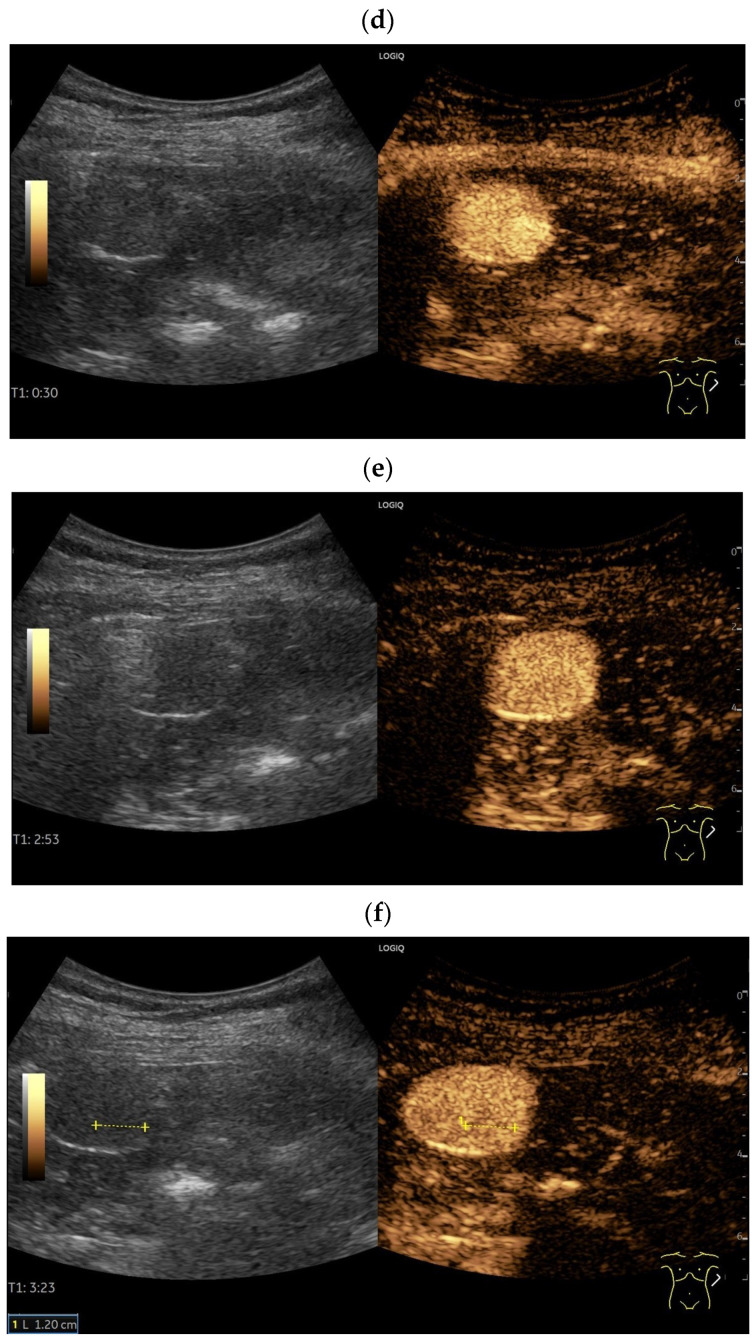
Splenosis with hemangioma. The patient had previously undergone a pancreatic tail resection with splenectomy for a neuroendocrine pancreatic tumor. During follow-up, a 30 mm round, smooth-bordered lesion (yellow arrow) was found in the former splenic bed. This lesion exhibited spleen parenchyma-like echogenicity but also included a 12 mm focal hypoechoic lesion (white arrow). Small vessels were visible on CDI at the periphery of the hypoechoic lesion (a). Contrast-enhanced ultrasound (CEUS) using 1.2 mL of SonoVue showed heterogeneous enhancement of the mass in the arterial phase, while the hypoechoic lesion displayed smooth, ring-shaped enhancement (b). The enhancement of the mass became homogeneous (c). The small lesion showed centripetal enhancement with complete homogeneous hyperenhancement at 30 s (d). Subsequently, the small lesion’s enhancement leveled to match that of the surrounding parenchyma at 2:53 min (e). Enhancement of the mass remains uniformly intense, while the small lesion showed a faint subtle hypoenhancement (f). The finding was classified as splenosis with a small hemangioma. Hyperenhancement was present for over 5 min.
